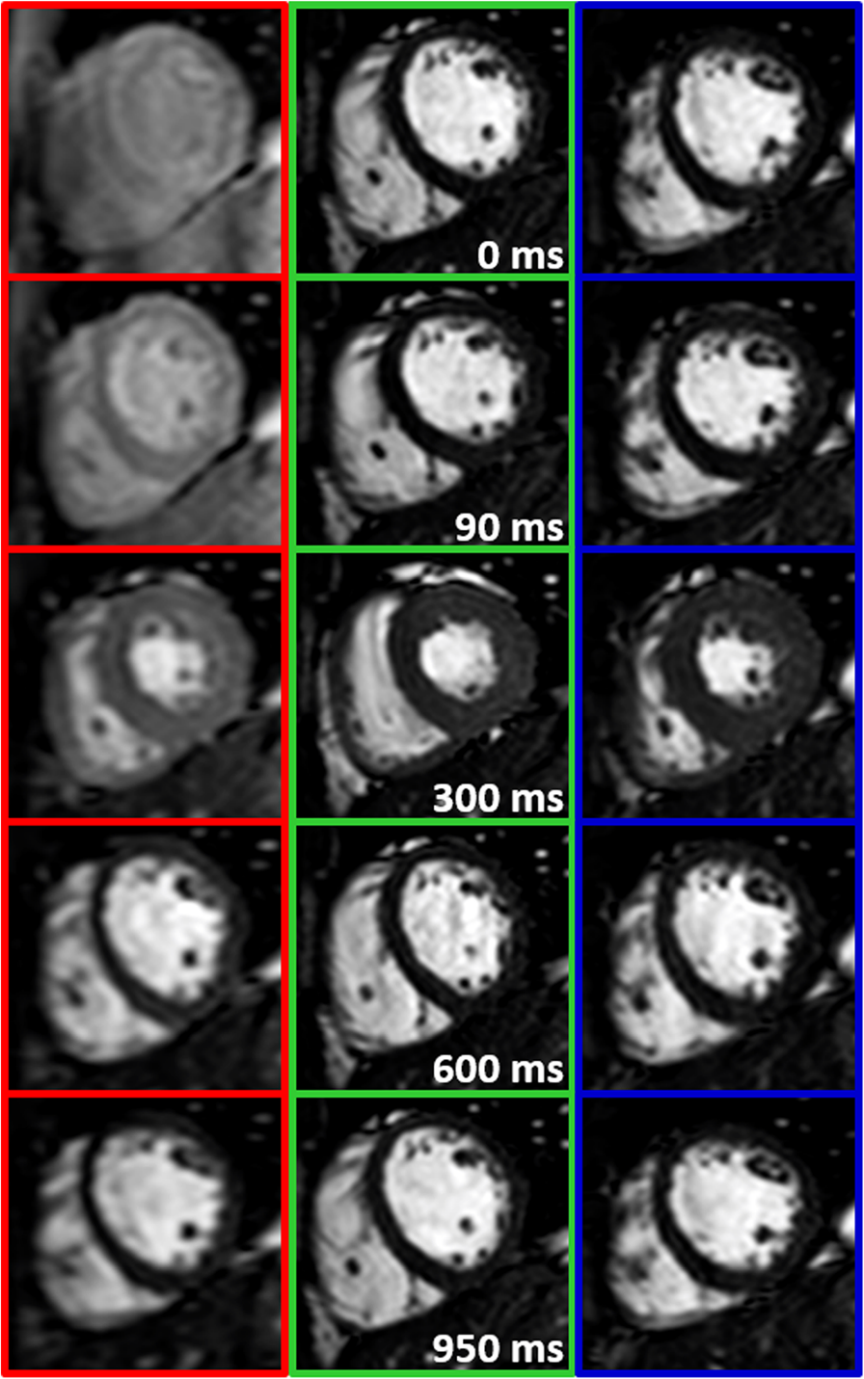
Representative cardiac-gated balanced steady-state free precession (bSSFP) images from healthy subject 3 with no steady-state (SS) preparation for cardiac-triggered (CT) sequence (red), 1-RR SS preparation for breath-hold (BH) sequence (green), and SS preparation for respiratory-triggered (RT) sequence (blue). Unlike in the case of CT sequence, in the RT sequence, tissue magnetization attains steady-state in a manner analogous to the conventional BH sequence with 1 R-R preparation
